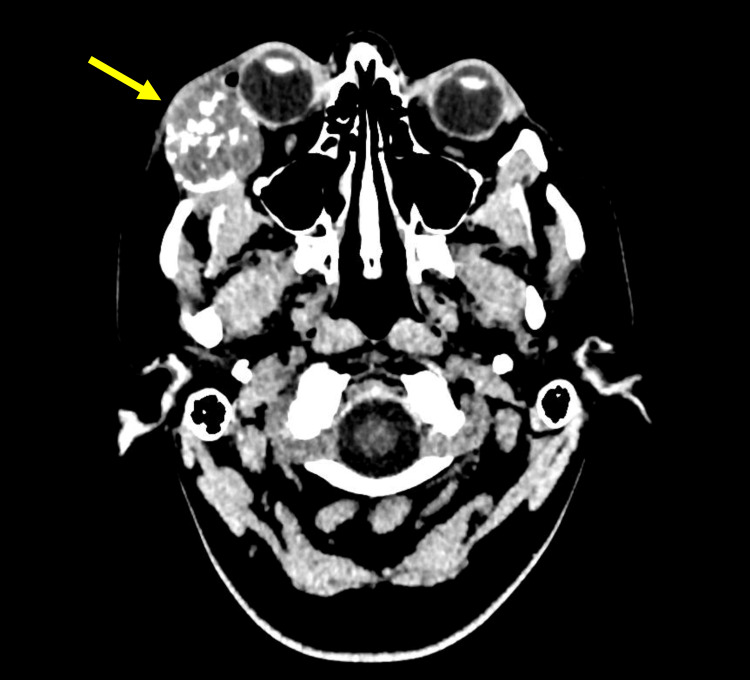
Axial view of the CT head demonstrating the bony lesion (indicated by the arrow) at the right lateral orbit
